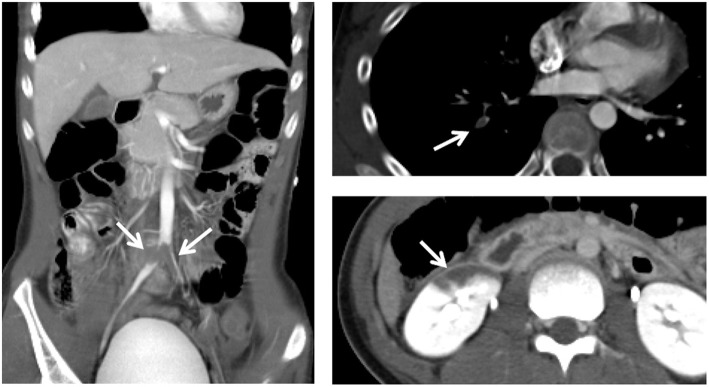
Contrast-enhanced computed tomography reveals multiple thrombi (arrows) in the bilateral common iliac, external iliac, and femoral arteries, right pulmonary artery, and right renal artery.
